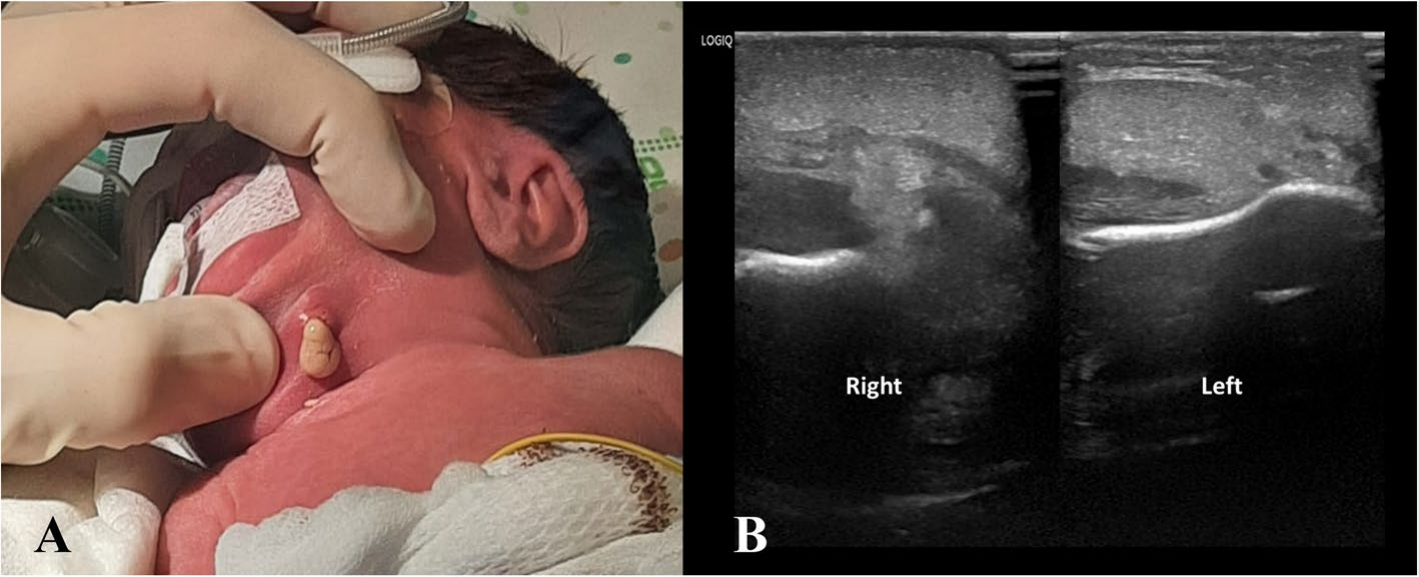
Case #2 drainage of abundant purulent material from the lesion sited in the right submandibular area (A). Ultrasound scan with the evidence of tissue inflammation extended up to the subfascial latero-cervical area in the right cervical side, compared to the normal left one (B)
